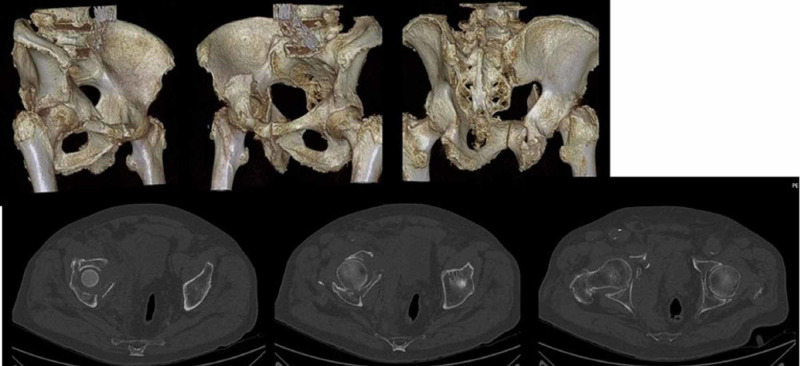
Preoperative CT and reconstruction of an 83-year-old patient with an acetabular fracture
